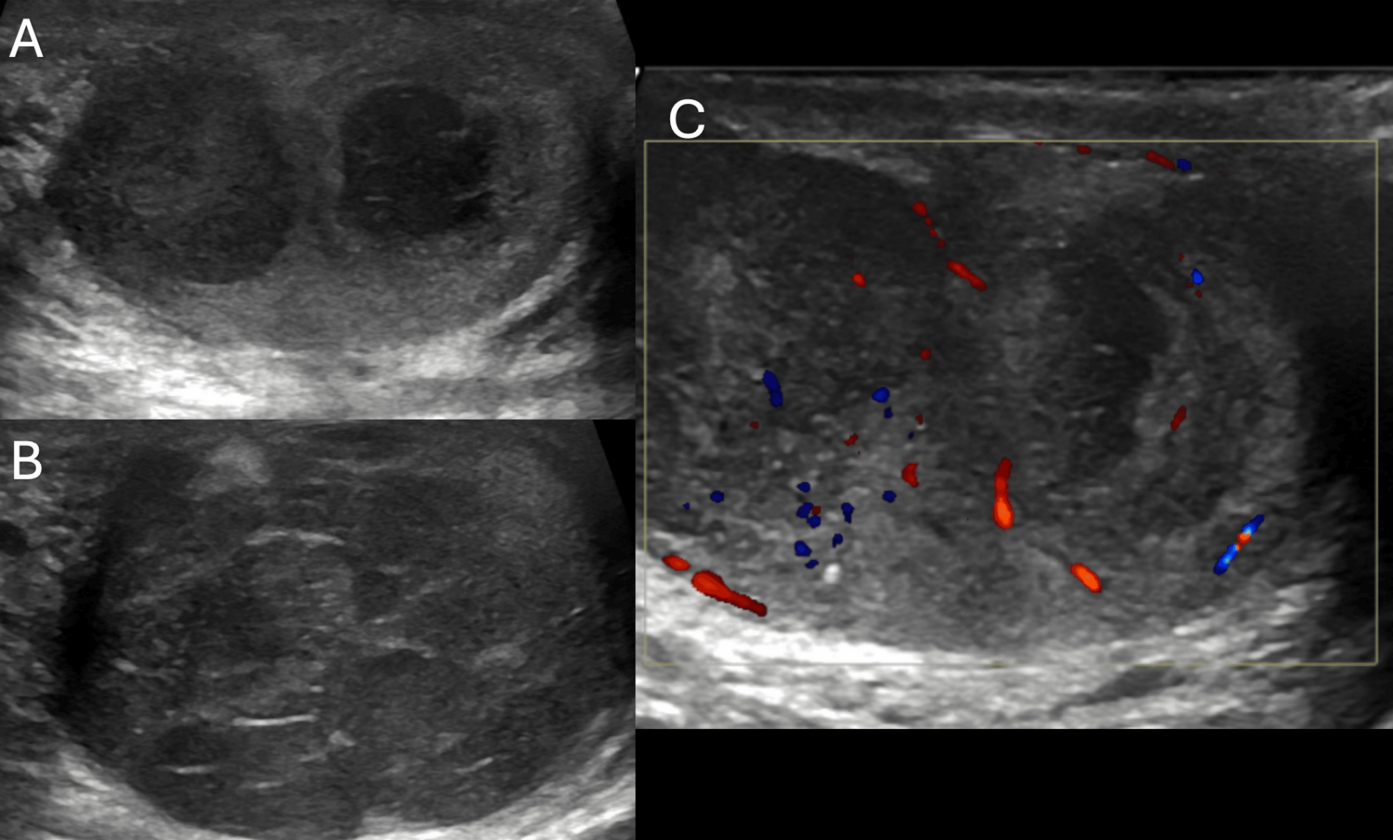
Testicular tumor Figures 5A, 5B show that the testis is replaced with diffuse heterogenous lesion, resulting in distortion of testicular architecture and enlarged appearance. Figure 5C shows areas of internal vascularity on color Doppler.
